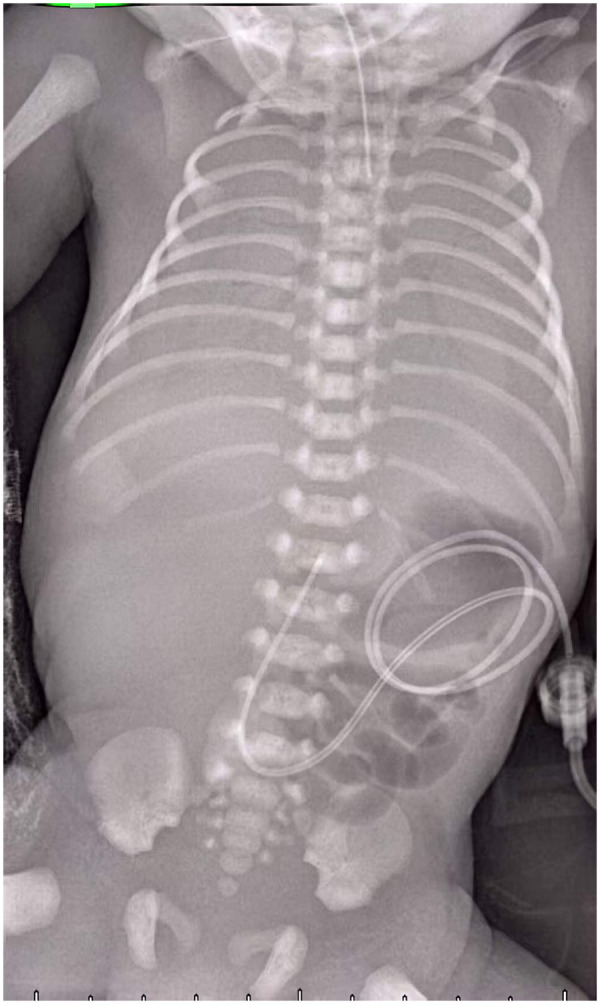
Anteroposterior chest-abdominal radiograph of the neonate showing an umbilical venous catheter and diffuse bilateral pulmonary opacification (“white lung”), consistent with severe neonatal respiratory distress syndrome.
